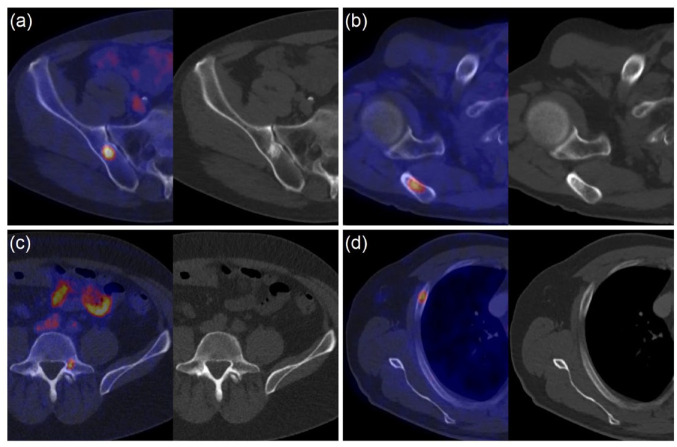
Four examples of lesions on fused PSMA PET/CT (left) and low-dose CT
